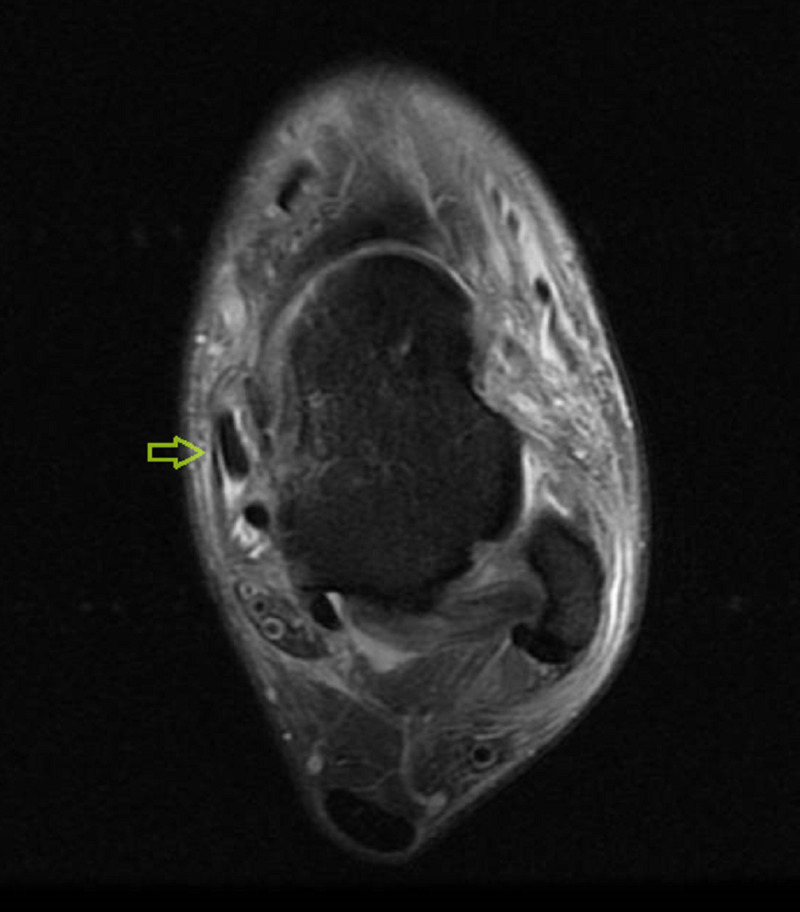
T2-weighted MRI (transverse plane) demonstrating an anterior subluxation of the tibialis posterior tendon over the medial malleolus (green arrow).
